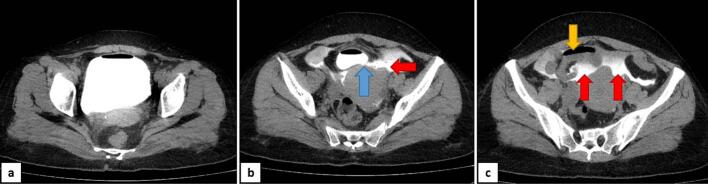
CT cystography a. the bladder with contrast agent b. bladder wall defect (blue arrow) and leakage of contrast (red arrows) c. small bowel loop (yellow arrow) and intraperitoneal contrast leakage (red arrows).
